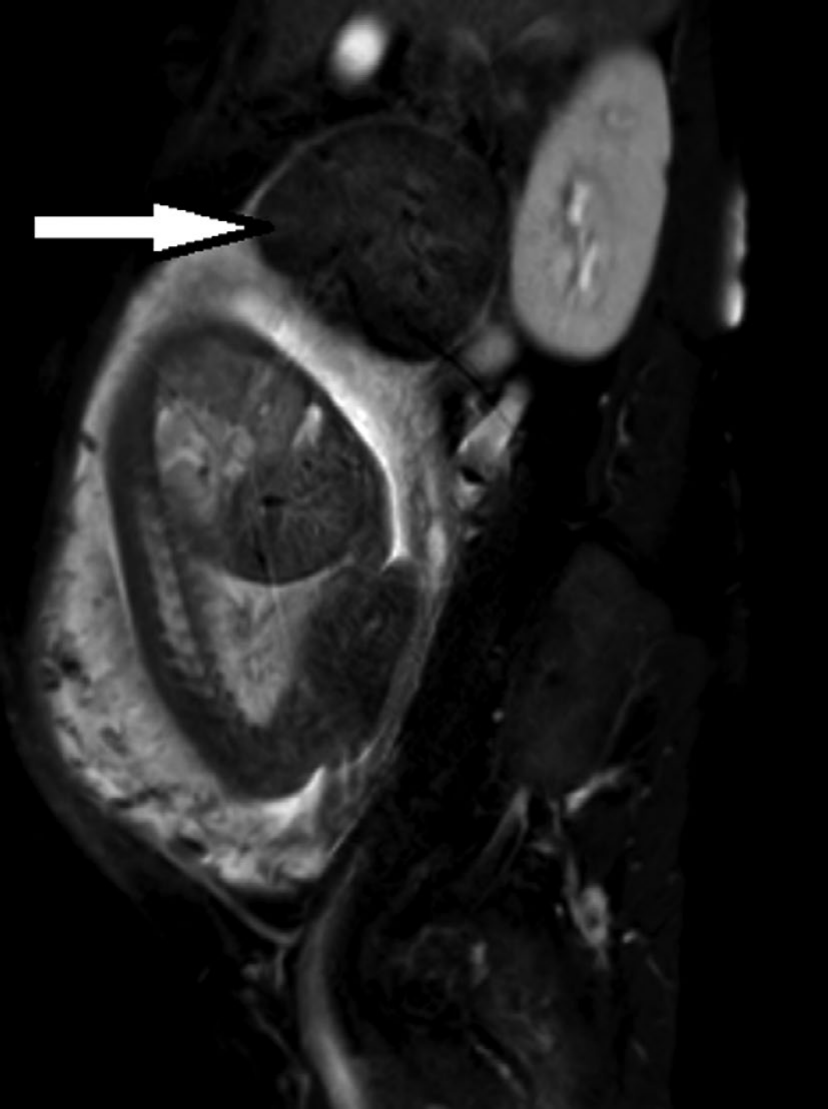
Magnetic resonance image at 34 wk of gestation (sagittal, T2‐weighted). The uterine fibroid (white arrow) is located on the maternal cranial side
